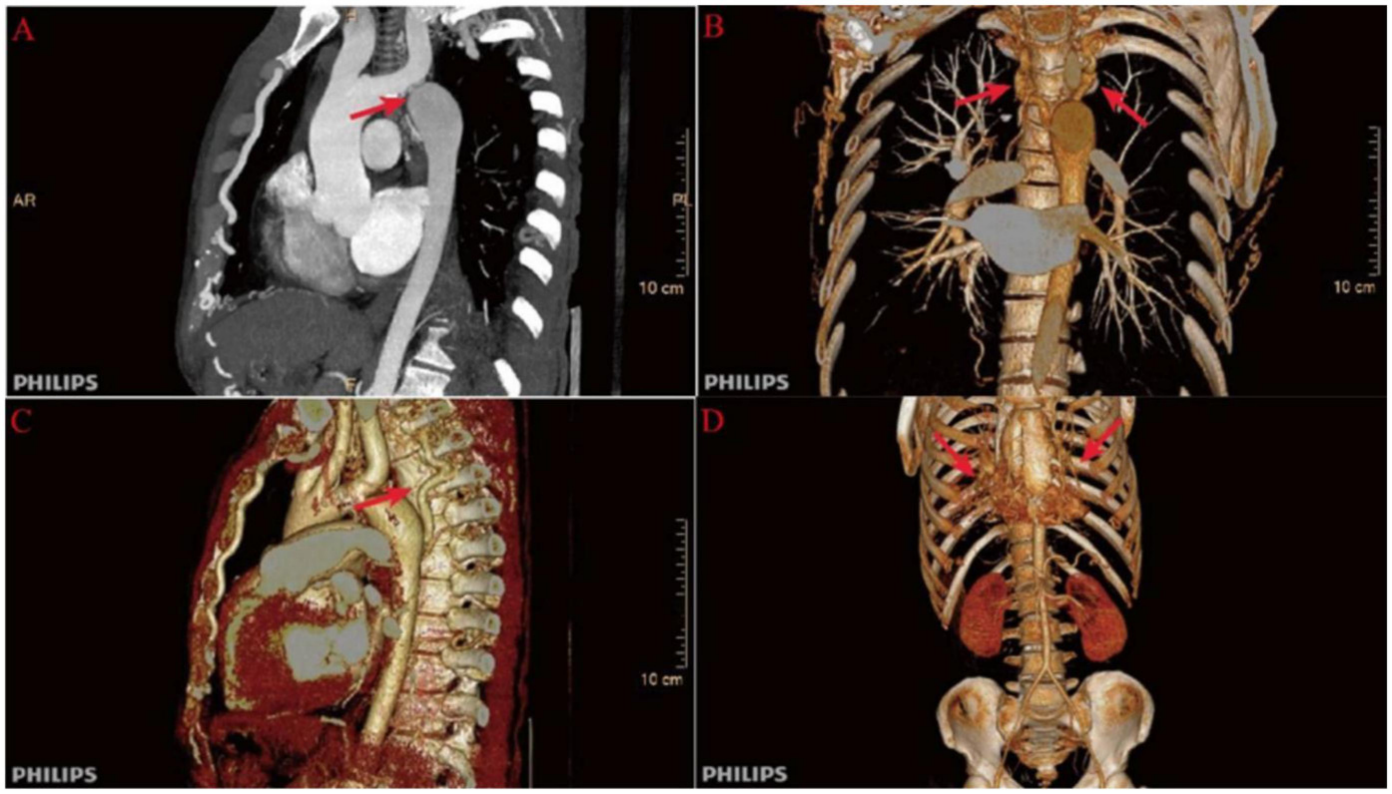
Preoperative computed tomography angiography (CTA) findings. (A) Severe narrowing at the aortic isthmus (“sandglass-shaped” coarctation, arrow); (B, C) Markedly dilated and tortuous paravertebral arteries, demonstrating extensive collateral circulation; (D) Prominent internal thoracic arteries serving as major collateral pathways.
